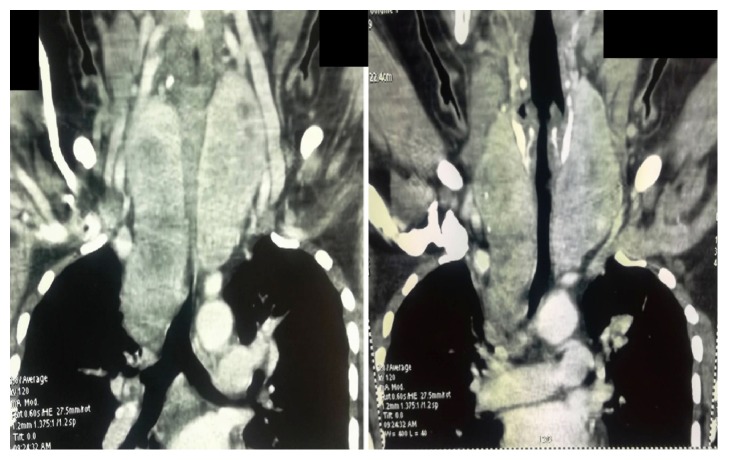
Cervical computed tomography scan revealed an enlarged plunging multinodular thyroid gland.
